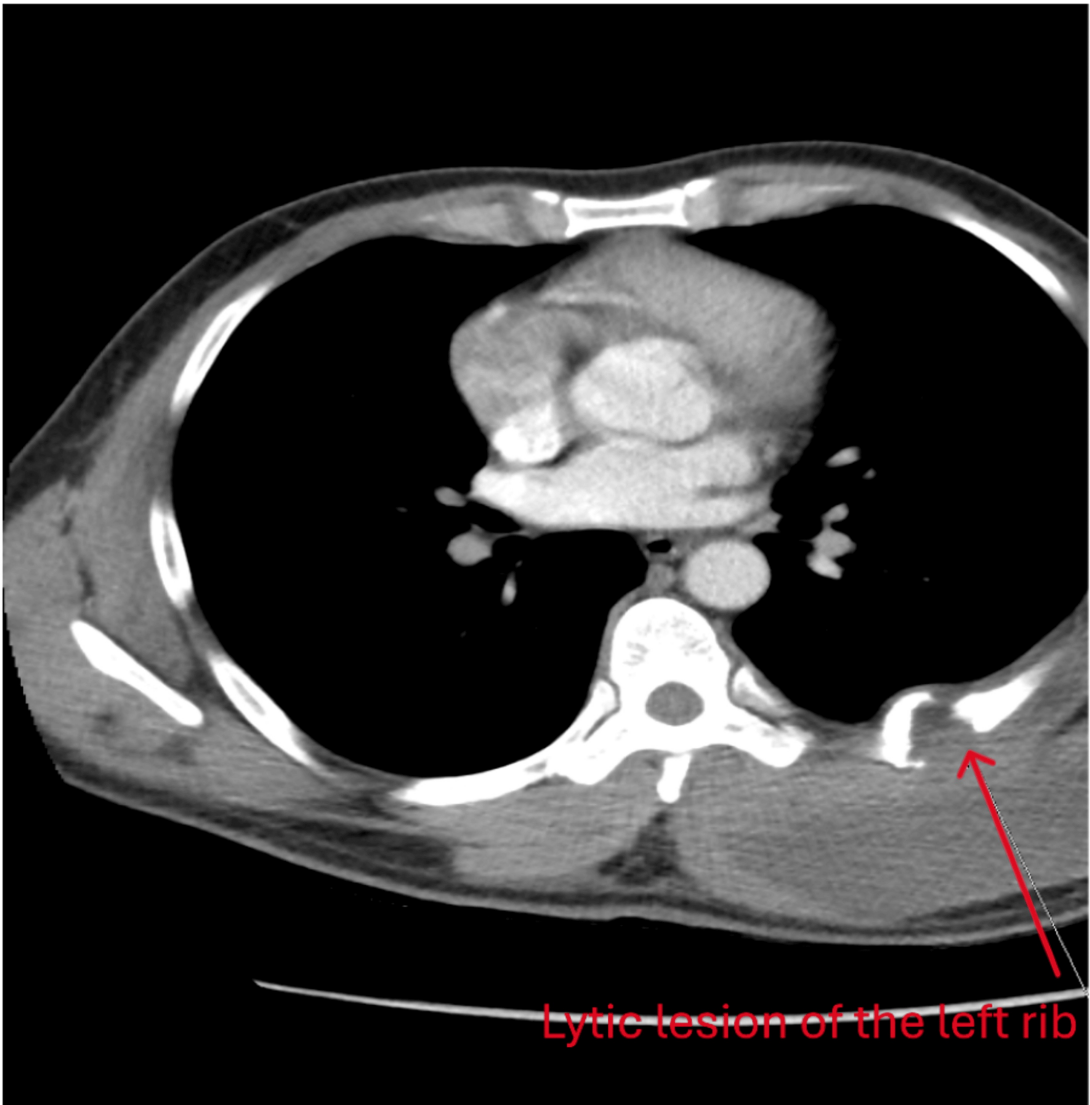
CT of the chest showing a lytic lesion of the rib due to TB
